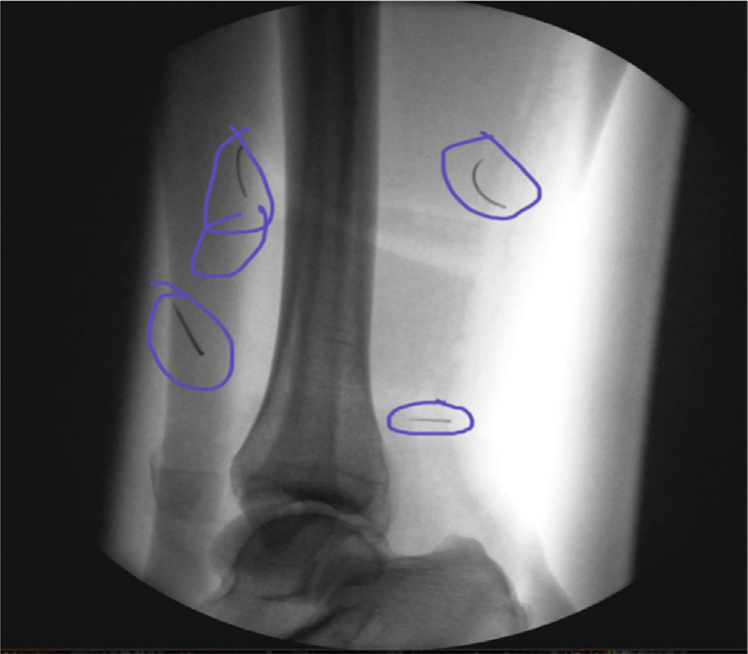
Needle location on radiograph of distal tibia.
